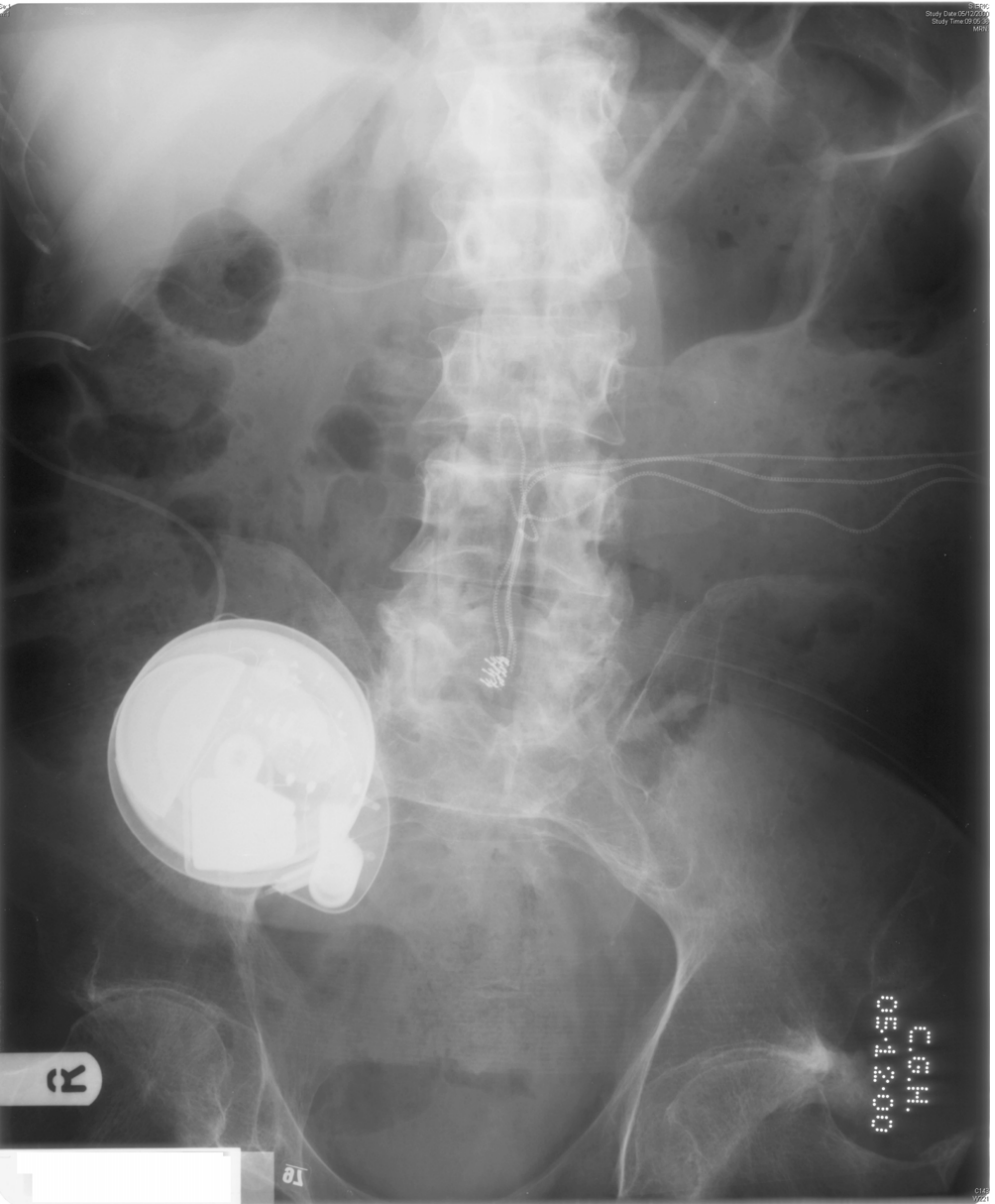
X-ray of abdomen, taken on 05 December 2000, shows baclofen pump on the right side. The electrodes of sacral anterior root stimulator can be seen in midline in the lumbar area. The wires for the sacral anterior root stimulator travel to the left side of abdomen where the receiver is located.
